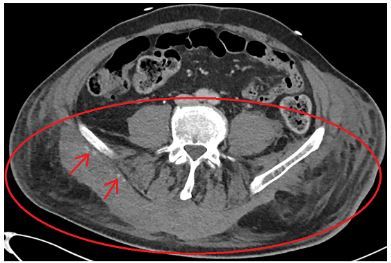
Computed tomography of the abdomen eight hours after admission with a Morel-Lavallée lesion in the lumbar region. A mass is visible on the dorsal side of the pelvis. The oval shows the main lesion. The arrows indicate the contrast extravasation.
